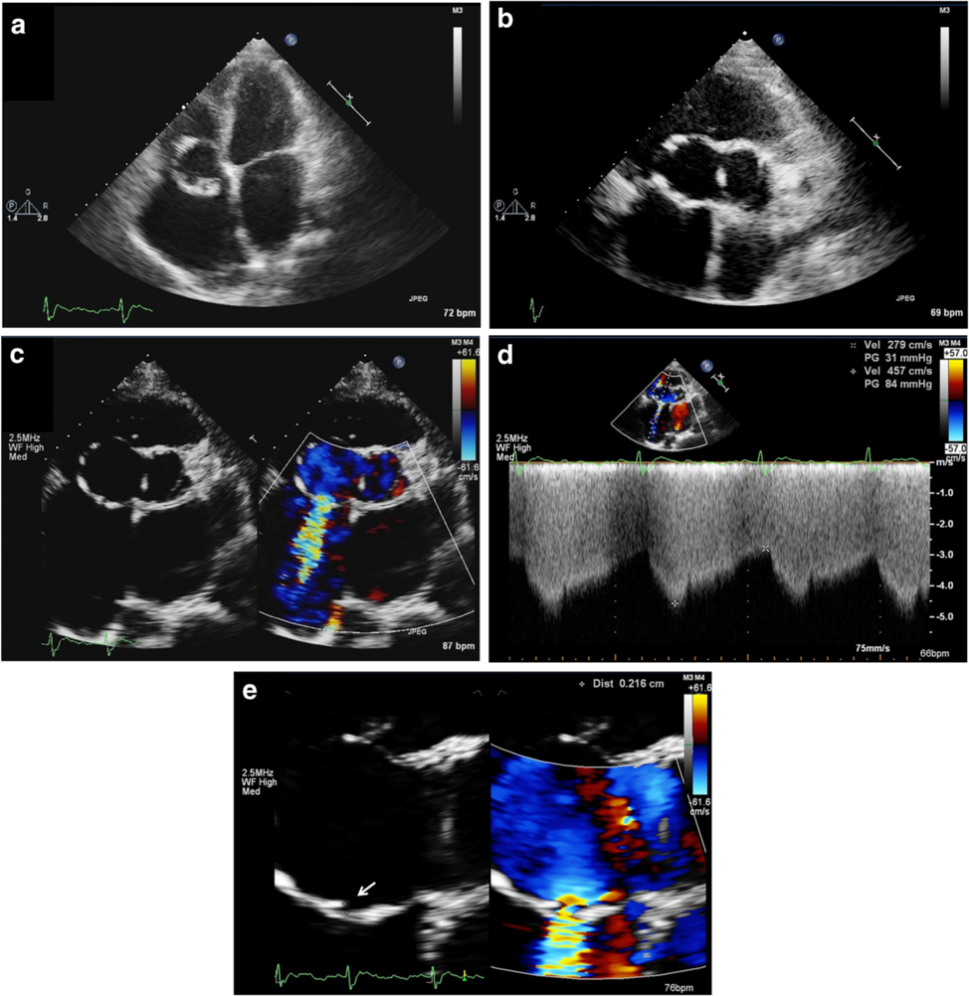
Transthoracic echocardiography of ruptured sinus of Valsalva aneurysm. a. Apical four chamber view of transthoracic echocardiography reveals an aneurysm with thick and hyperechoic wall at the level of tricuspid ring. b. Parasternal short-axis view shows bicuspid aortic valve and an aneurysm (SVA) protruding from right coronary cusp to the right atrium. c. In the same view, color Doppler image shows shunting from SVA into right atrium. d. Continuous wave Doppler demonstrated the continuous flow. e. Zoom-in view from figure 1c revealed the defect (*arrow*) at the posterior aspect of the SVA. SVA indicates sinus Valsalva aneurysm; RA, right atrium; Av, aortic valve; LA, left atrium.
